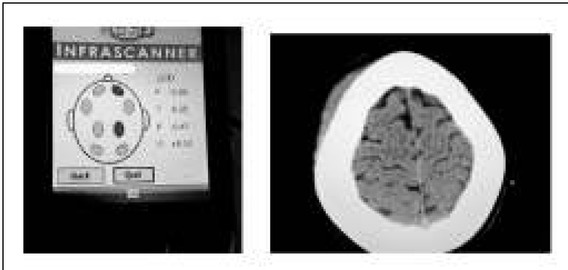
Wynik fałszywie dodatni. Infrasacnner wynik badania dodatni, TK-bez cech krwawienia wewnątrzczaszkowego, jedynie krwiak podczepcowy (materiał własny). Fig. 4. False positive result. Positive Infrascanner screening result. Head CT with no evidence of intracranial bleeding but only subgaleal haematoma (own material).
